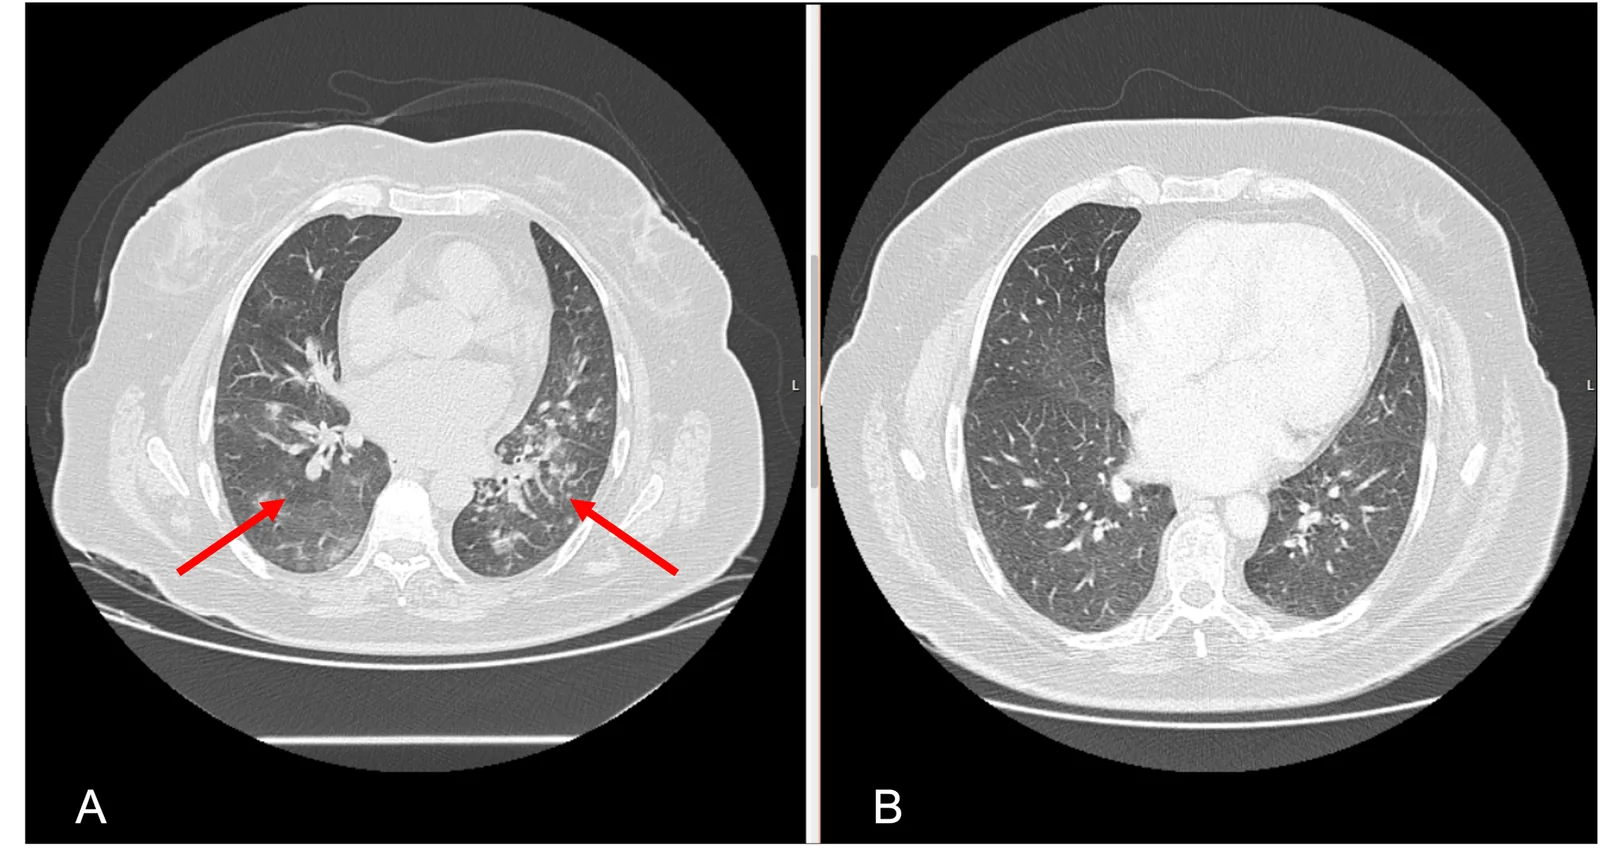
Chest CT findings at admission and follow-up. (A) Axial CT slice at admission showing bilateral ground-glass opacities (arrows), predominantly perihilar. (B) Follow-up CT after targeted treatment, showing complete resolution of the previous findings. CT: computed tomography
